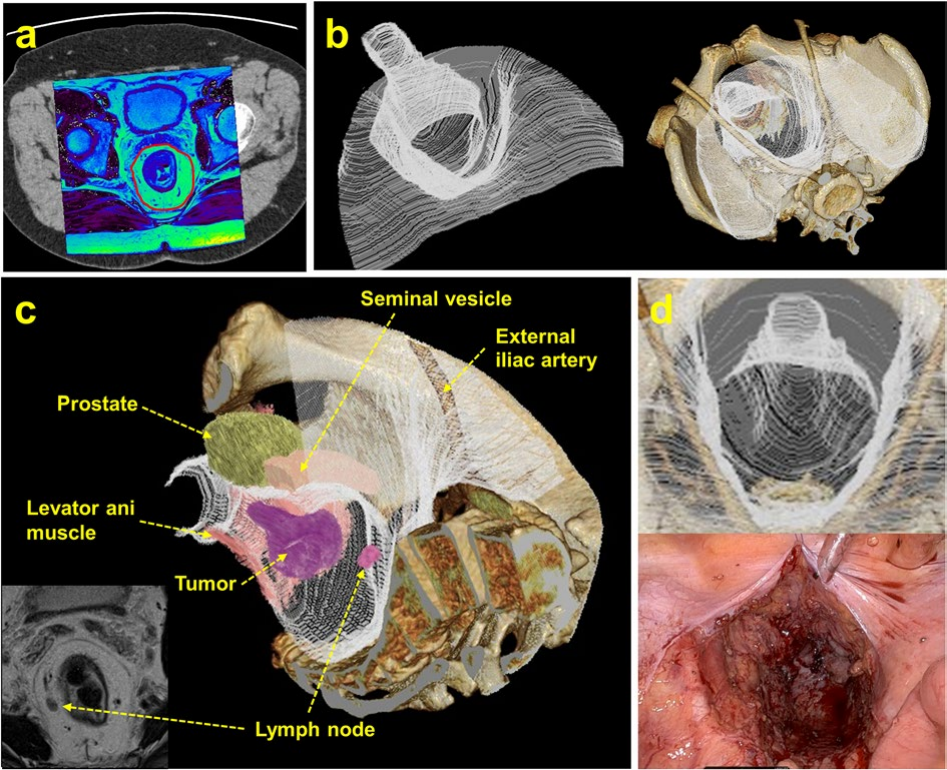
Three-dimensional (3D) visualization of the total mesorectal excision (TME) dissection plane. (a) A T2-weighted magnetic resonance image was superimposed on the contrast-enhanced computed tomography image. The line of TME dissection was manually traced (red line). (b) Created 3D images of the TME dissection plane. (c) A 3D image of the TME dissection plane in a male patient. The primary tumor, swollen lymph node, pelvic bone, external iliac artery, levator ani muscle, prostate, and seminal vesicle are also shown. (d) Comparison of the 3D TME dissection plane image and intraoperative image of the pelvic cavity after TME and resection of the rectum in the same patient.
